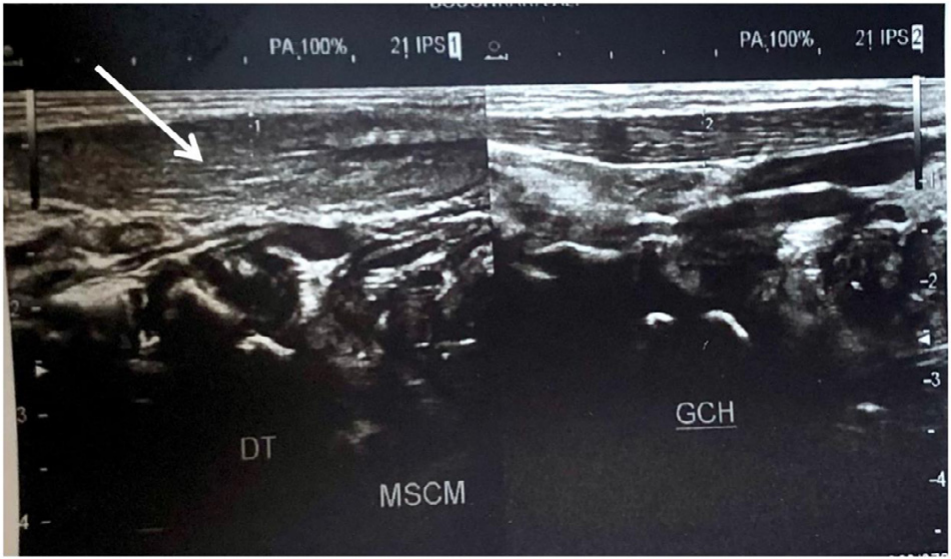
Ultrasound appearance of homogeneous fusiform hypertrophy of the right SCM muscle (white arrow) compared to the left SCM muscle.
